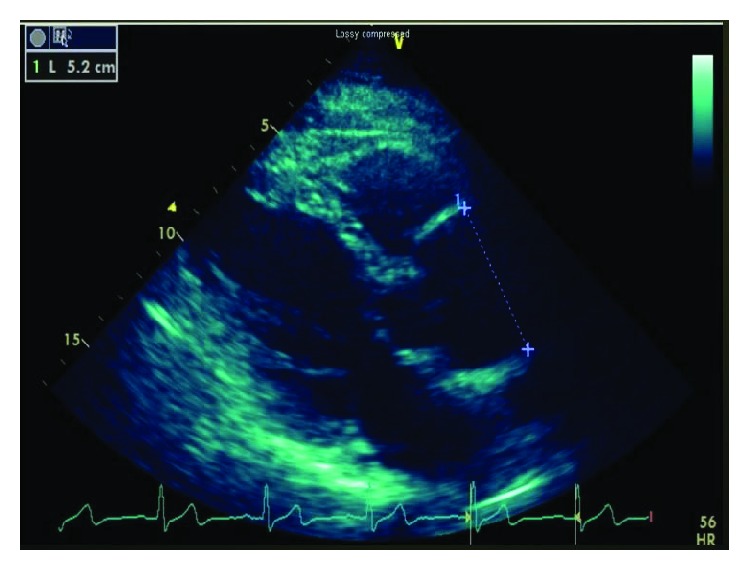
A parasternal long axis view on transthoracic echocardiography demonstrating an enlarging aortic root at 5.2 cm.
